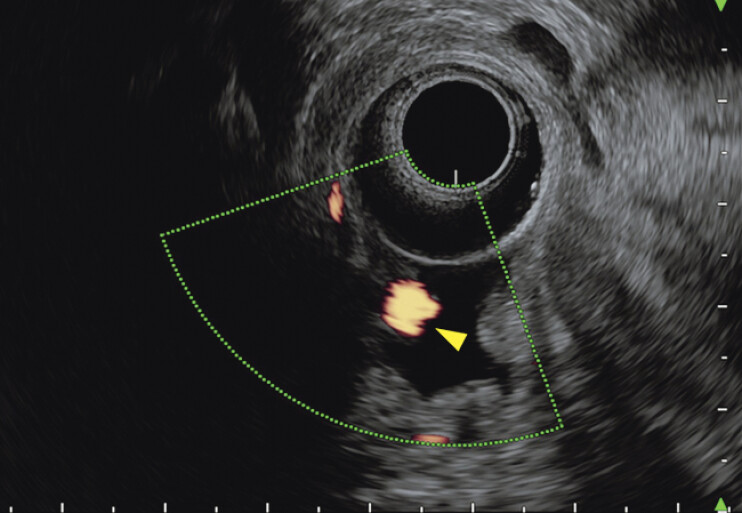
Doppler echography of endoscopic ultrasound (arrowhead) revealed the duodenal papilla with strong pulsation.
